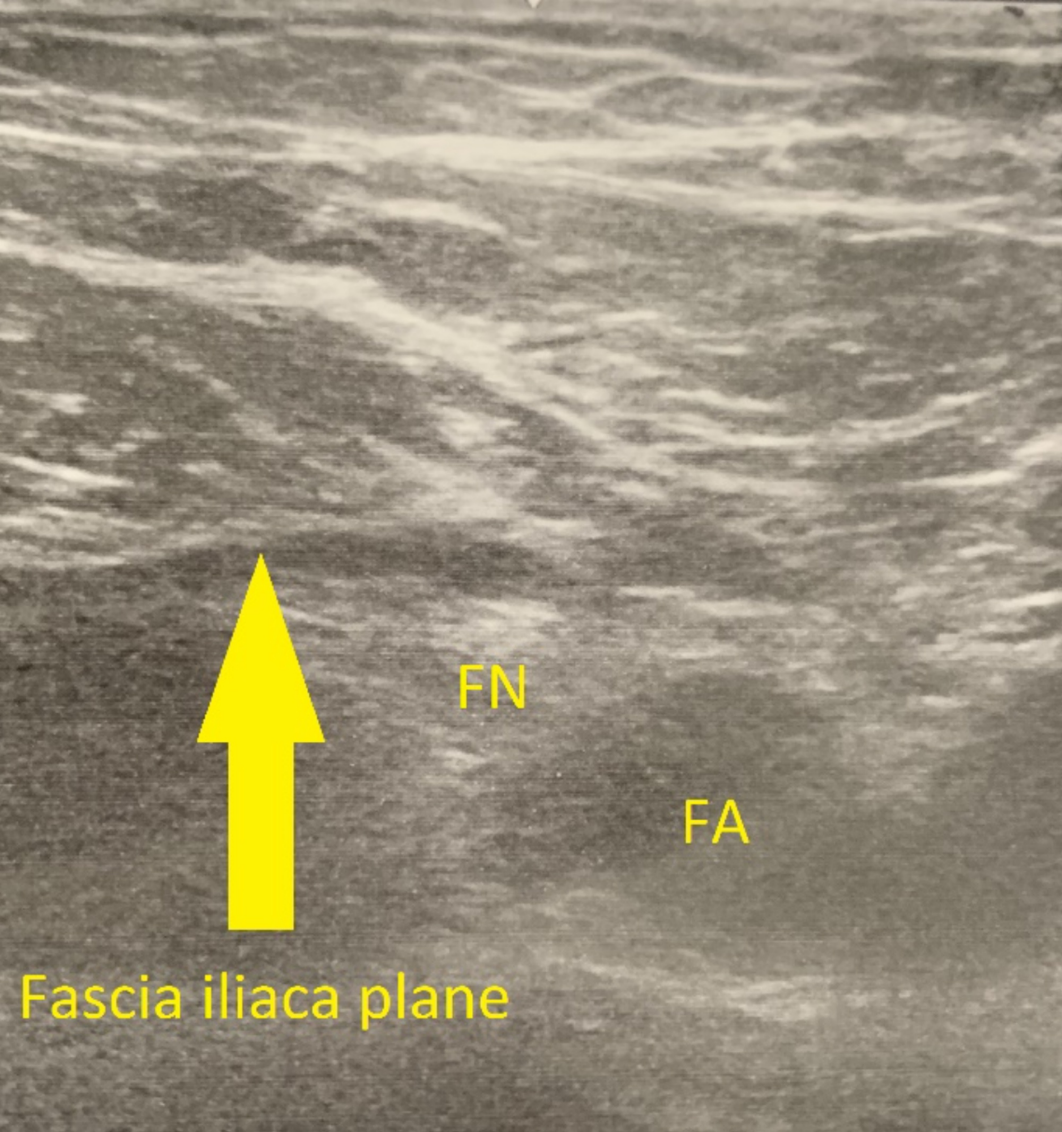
Ultrasound image of the iliacus muscle and fascia iliaca plane (yellow arrow), FN, and FA. FN, femoral nerve; FA, femoral artery
